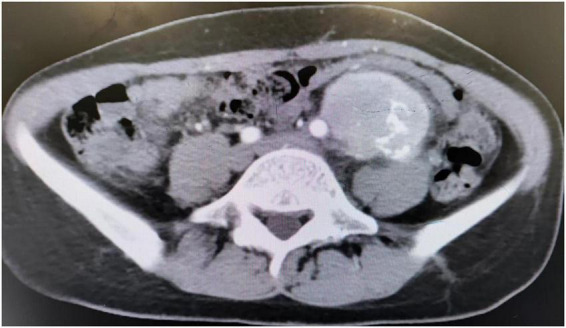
CT scan showed an abdominal mass.
